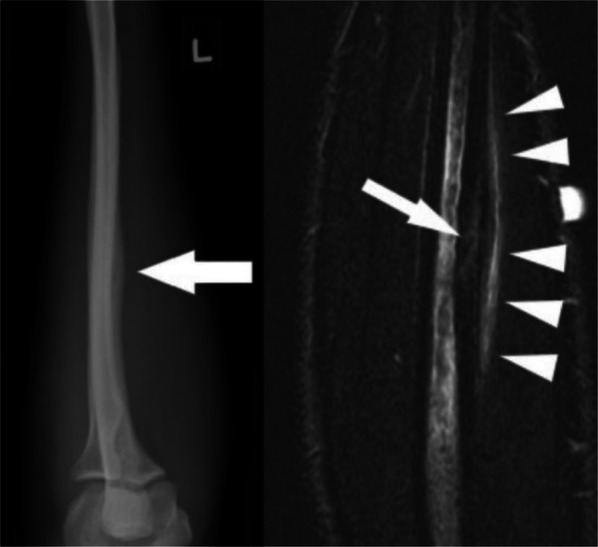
Ulnar stress fracture. (Left) Lateral radiograph of a left forearm showing cortical thickening along the volar cortex; (Right) Sagittal inversion recovery image of a forearm demonstrating periosteal, cortical and medullary edema (arrowheads) along the volar aspect of the ulnar diaphysis as well as a subtle oblique low signal fracture line (arrow). Reproduced from Dean et al. [51], with permission
